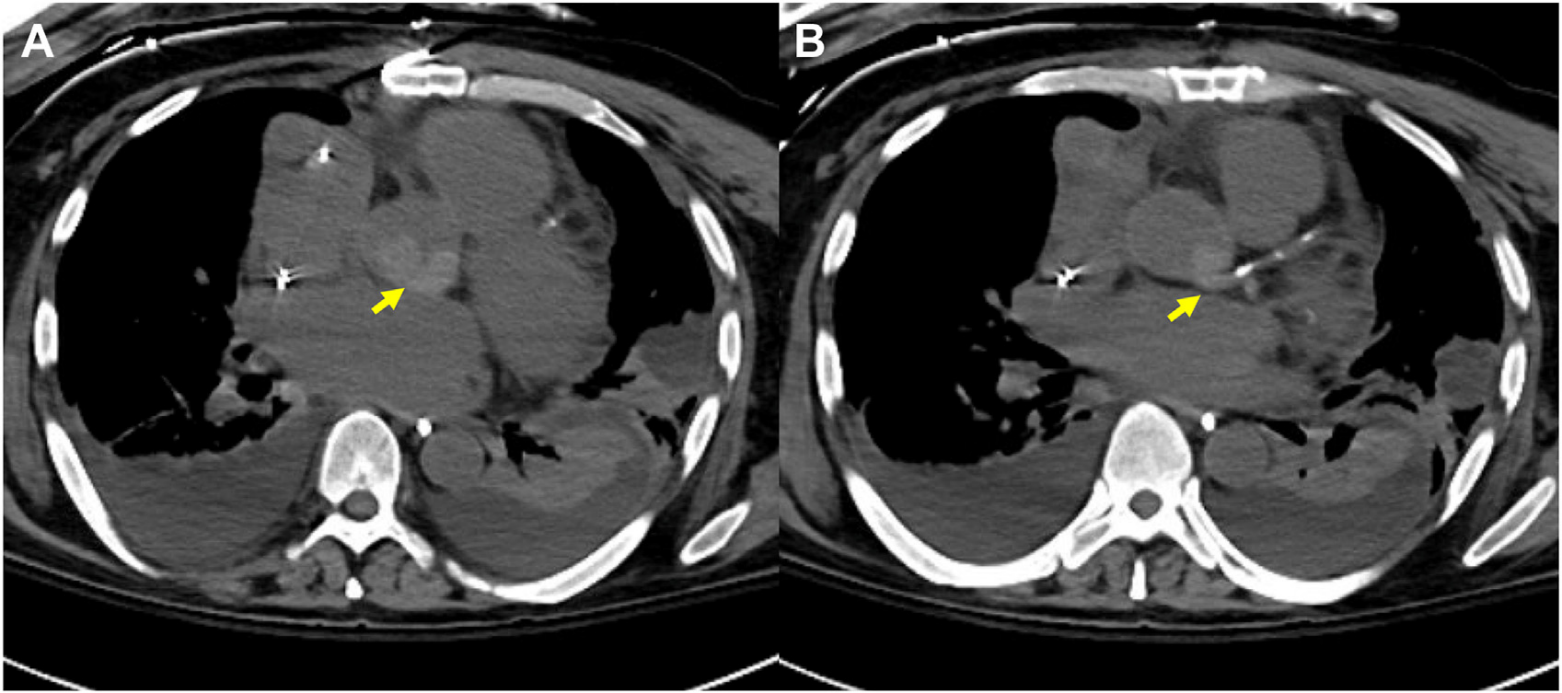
Computed tomography imaging demonstrating (A) a high-density 2.7 × 1.6-cm material within the aortic root (arrow), (B) extending into the proximal left main coronary artery (arrow).
